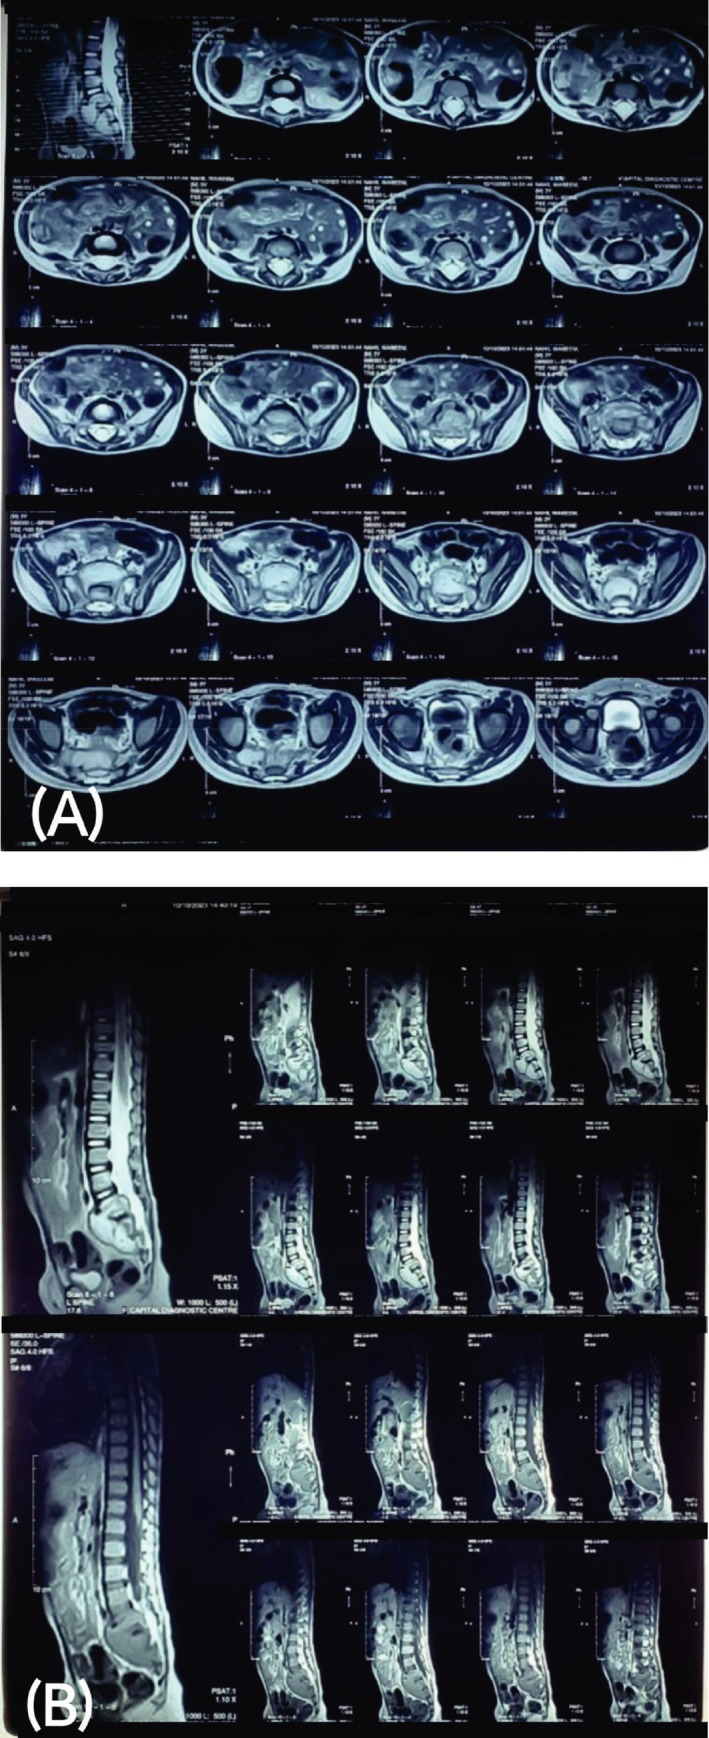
(A, B) Multi‐planar, multi‐sequence, non‐contrast enhanced magnetic resonance imaging of the lumbar spine showing a cold abscess in the pre‐sacral region of S1–S2 with intervertebral disc involvement. (A) Axial T2‐weighted MR images reveal a well‐defined, hyperintense lesion anterior to the S1–S2 vertebral bodies, consistent with a fluid‐containing cold abscess in the presacral space. The lesion causes anterior displacement of adjacent pelvic structures. The surrounding vertebral bodies show altered marrow signal intensity, and there is loss of normal disc height and signal at S1–S2, indicating discitis. (B) Sagittal and coronal T2‐weighted MR images confirm the presence of the presacral abscess tracking along the anterior aspect of the sacrum. The lesion appears hyperintense on T2WI and hypointense on T1WI, with irregular margins. The S1–S2 intervertebral disc shows signs of involvement, including reduced disc height and signal intensity, suggesting disc space infection (discitis). Vertebral endplate irregularity and bone marrow edema are also noted, which are consistent with spondylodiscitis.
